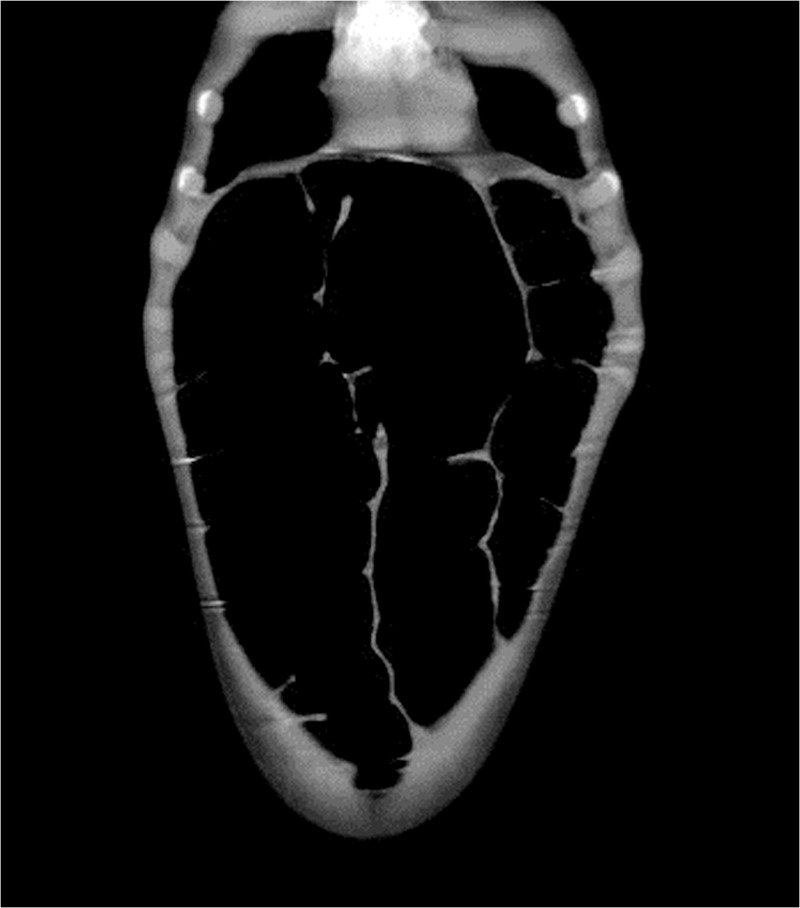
Sigmoid colon overlapping the liver and extended cephalad to the transverse colon in abdominal CT.
